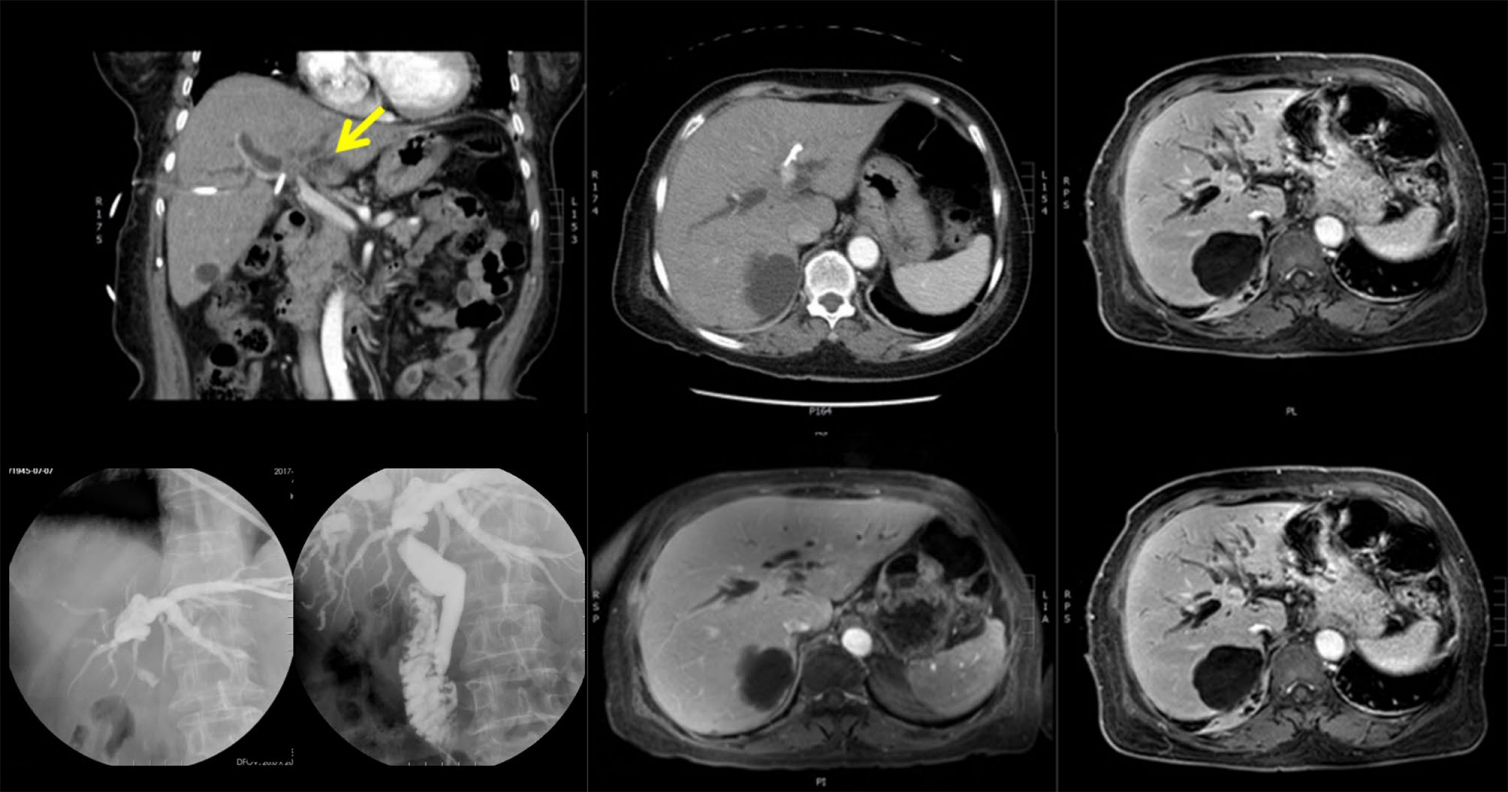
An index patient with unresectable hilar cholangiocarcinoma who underwent IRE treatment. Pre-operative contrast-enhanced abdominal CT/MR image showed hilar tumor with dilated IHD (upper part), whereas post-IRE treatment at 3 months (middle lower part) and 28 months (right lower part) showed good local control (progressive shrinkage of tumor without any contrast-enhancing lesion) with complete response. Trans-catheter cholangiography by injecting contrast medium through the PTCD tube also showed satisfactory results (left lower part). Before IRE treatment, an obstructive bile duct was observed (left), but three months post-IRE treatment, a patent bile duct the (right).
